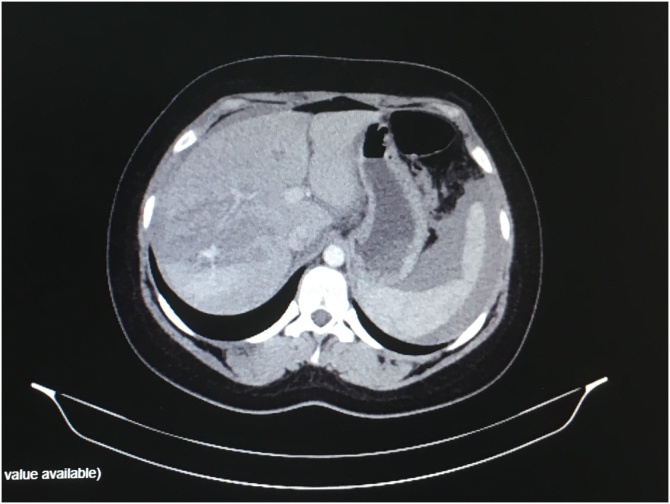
Spiral Computed tomography of the abdomen and retroperitoneal space revealed hemoperitoneum and hepatic parenchyma rupture.
